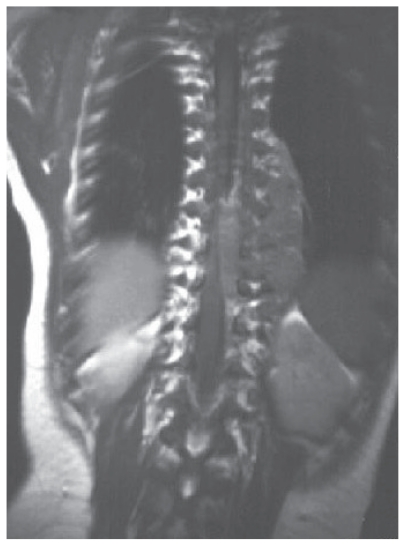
Coronal T1-weighted image demonstrating a large, left, paraspinal neuroblastoma in the mid/lower thoracic region.
